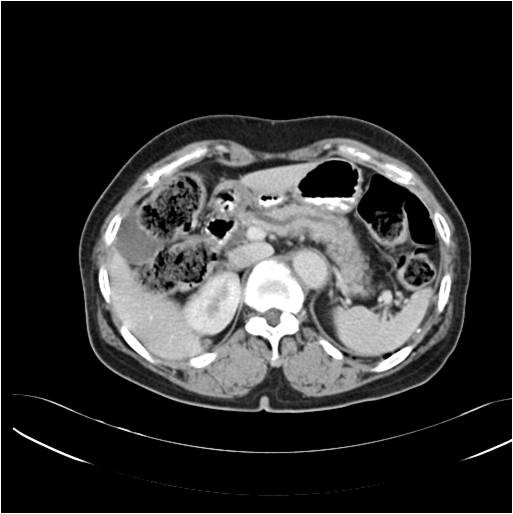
Abdominal CT finding. The pancreatic duct was diffusely dilated without a definite obstructive mass lesion.
